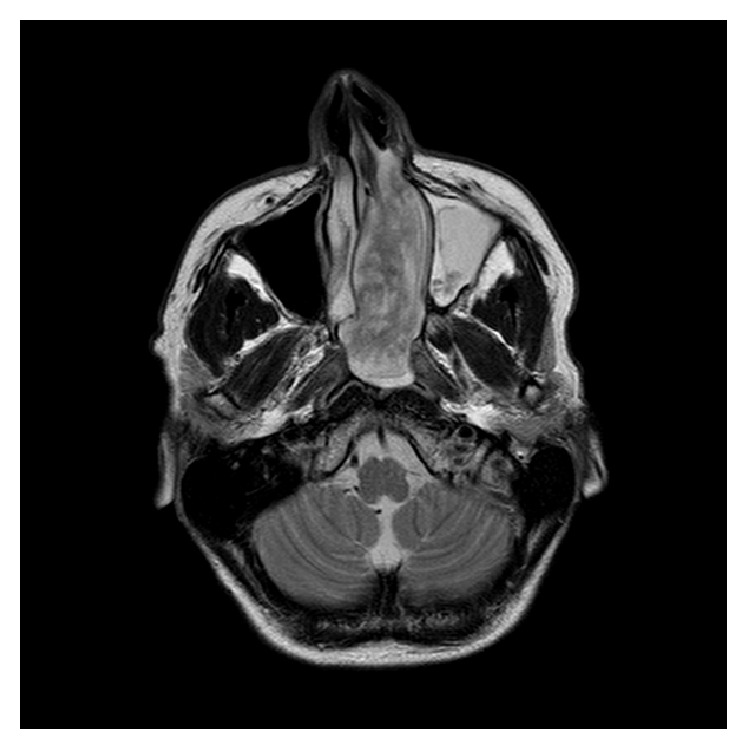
MRI (axial, T2 weighted) showing tumour involving the postnasal space, nasopharynx, and ethmoid air cells and secretions filling the left maxillary antrum and frontal sinus.
